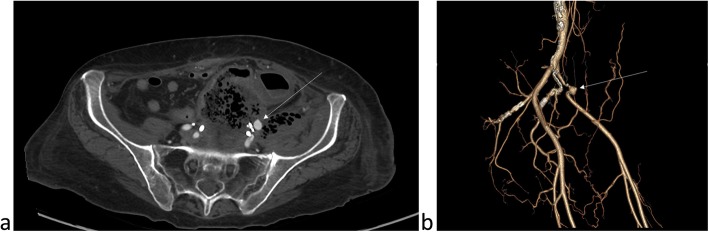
An a axial cut of a Computed Tomography Angiogram and b a 3D reconstruction showing the pseudoaneurysm (arrow) arising from the left external iliac artery with narrowing of the vessel proximal and distal to the pseudoaneurysm appreciated on the 3D reconstruction, which are related to surgical clips
